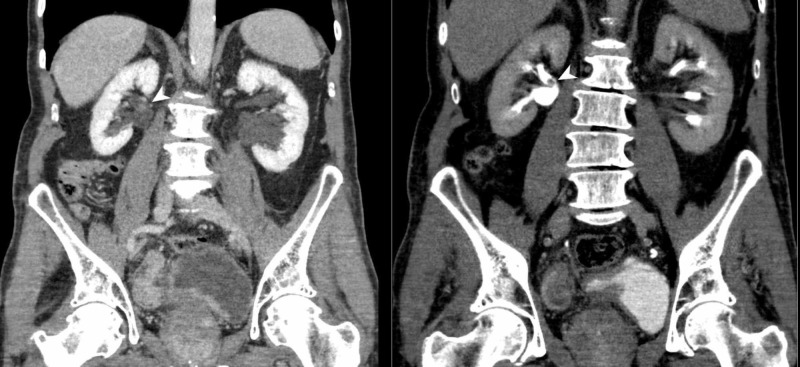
Bladder and renal TCC (CTU) CT urography (coronal reformat) demonstrates irregular urinary bladder wall thickening and also a sessile polypoid filling defect in the posterior aspect of the right renal pelvis (arrow heads). Findings are suggestive of multifocal urinary tract transitional cell carcinoma (TCC).
